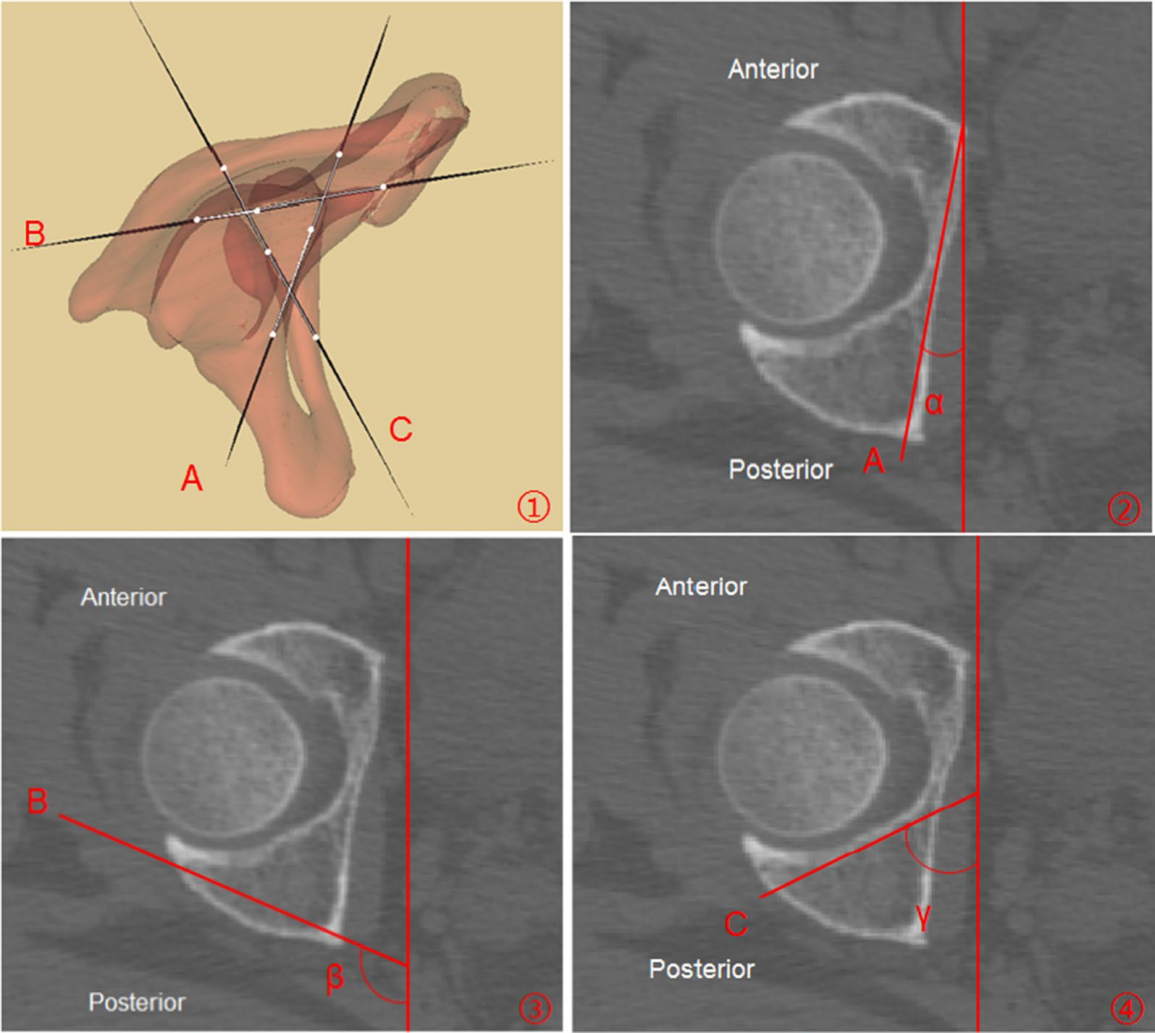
Measurement of angles between the sagittal plane and the three lateral faces of the triangular prism. ① Three lateral faces of the triangular prism: A: Quadrilateral plate; B: Posterior acetabular wall; C: Acetabular dome (According to the relevant anatomical measurements [3], the minimum cross section of the acetabular posterior column is mostly located at the level of the middle part of the acetabulum; therefore, at this level, the axial CT images were chosen to be measured.); ② A: Quadrilateral plate; α: Angle between quadrilateral plate and sagittal plane; ③ B: Posterior acetabular wall; β: Angle between posterior acetabular wall and sagittal plane; ④ C: Acetabular dome; γ: Angle between acetabulum and sagittal plane
